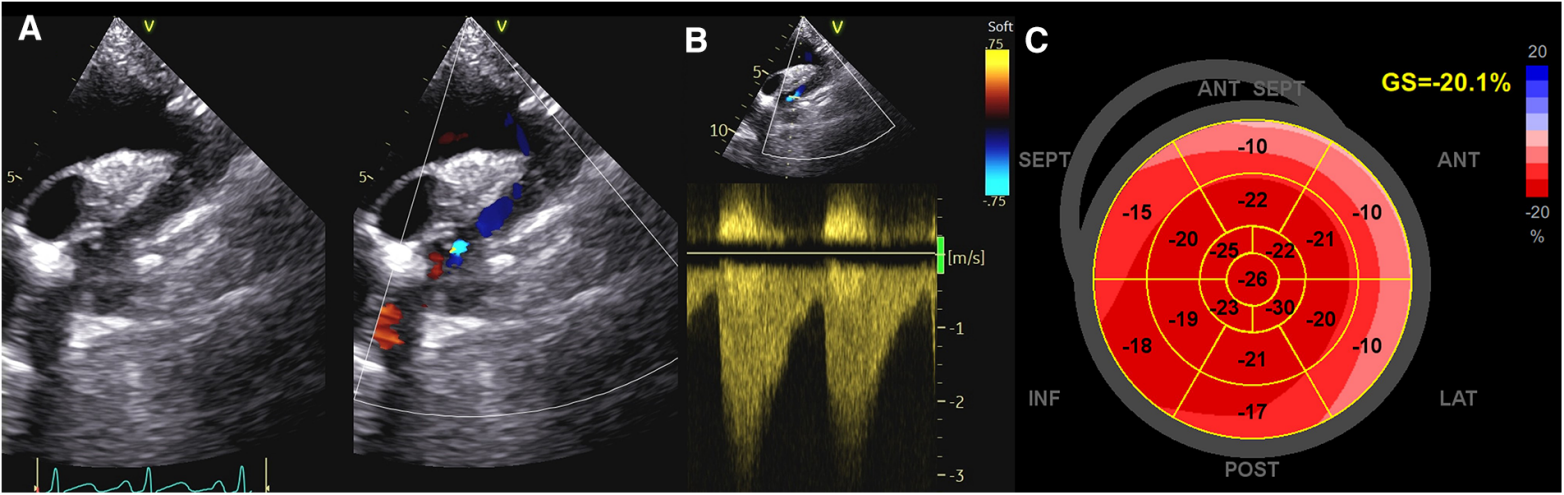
Evaluation of systolic function in a patient with coarctation of the aorta. (A,B) CoA as assessed by Color and CW Doppler; (C) GLS values in the same patient. As noticed, strain values are reduced in basal segments, which are impaired also in hypertensive patients. CoA, coarctation of the aorta; CW, continuous Doppler; GLS, global longitudinal strain.
